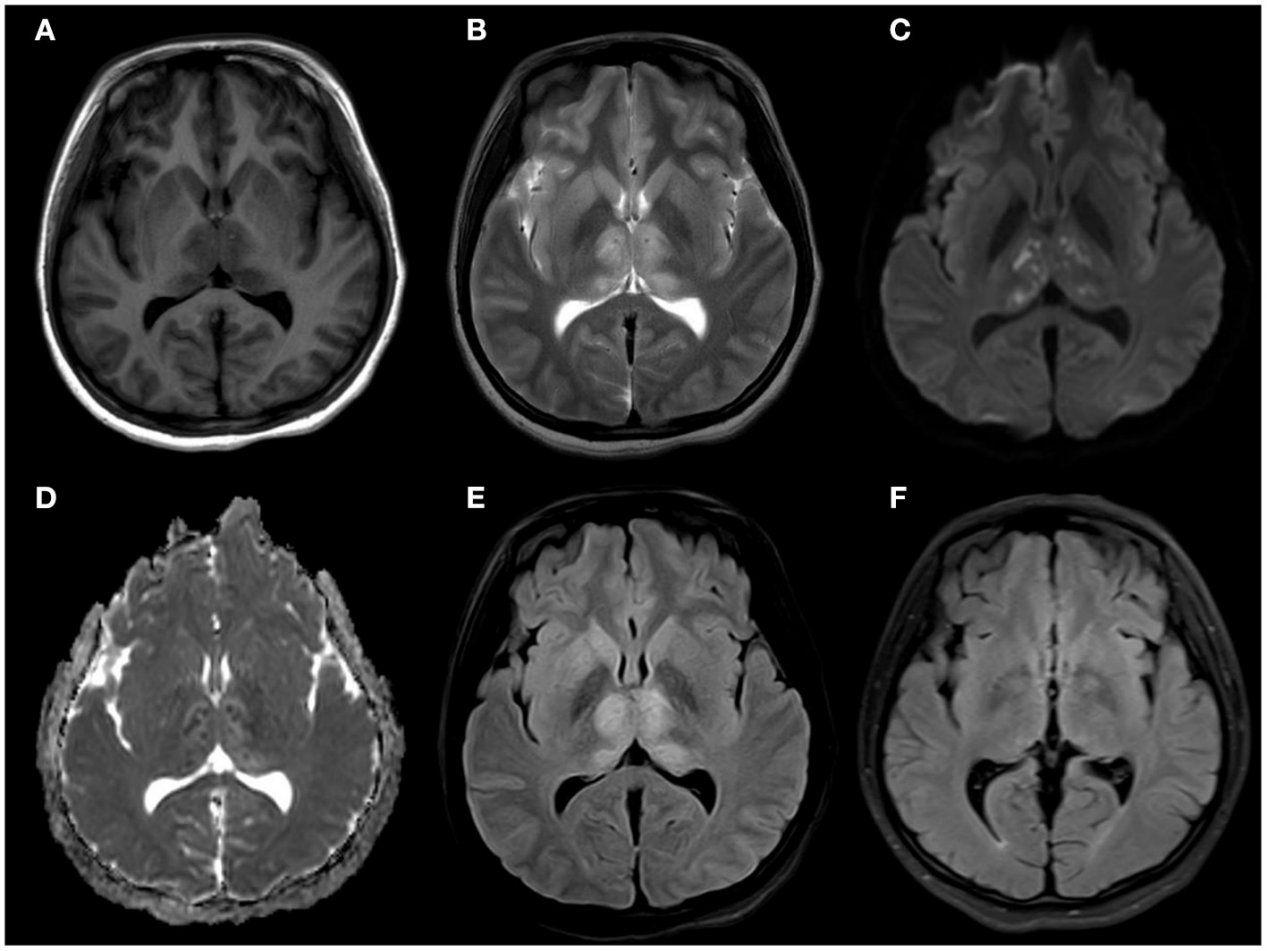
(A) MRI brain axial T1: slightly hypointensity in the symmetric thalamus, head of caudate nucleus, and right lenticular nucleus (B) MRI brain axial T2: hyperintensity in the symmetric hippocampus, thalamus, head of caudate nucleus, and right lenticular nucleus. (C,D) MRI brain axial diffusion-weighted imaging: isointensity bilateral punctate hyperintensity in the symmetric thalamus, and showed hypointensity in ADC. (E) MRI brain axial T2 fluid-attenuated inversion recovery: slightly hyperintensity in the symmetric thalamus, head of caudate nucleus, no mass effect. (F) MRI brain axial T2 fluid-attenuated inversion recovery: The lesions had disappeared completely after 2 months.
